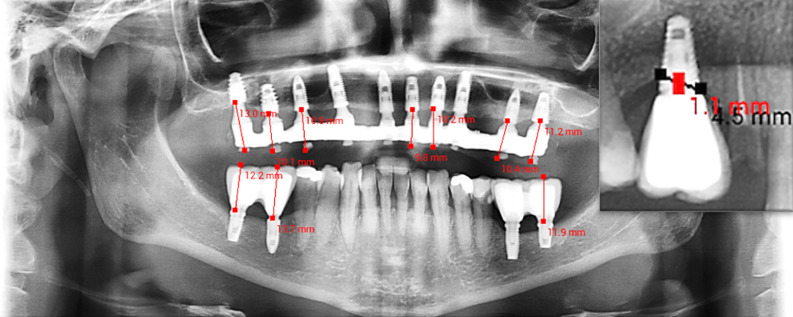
Panoramic radiograph with measurements for the height of the prosthetic rehabilitation of short and extra-short implants, and measurement of the peri-implant bone loss.
